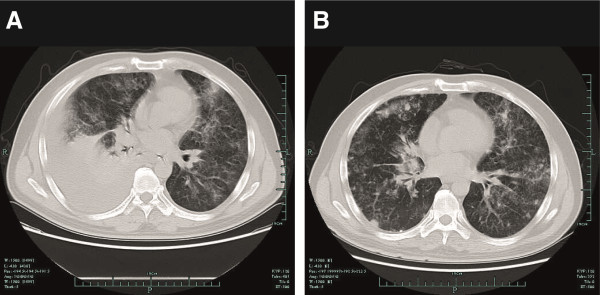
Computed tomography scans of the lungs. (A) Soon after the appearance of the patient’s respiratory symptoms, a computed tomography (CT) scan revealed septal thickening of the peribronchovascular interstitium, pleural effusion, segmental atelectasis in the right lower lobe of the lung and several enlarged lymph nodes in the mediastinum. (B) Discontinuation of anti-infection therapy, and 5 days after thoracentesis, extensive involvement of the parenchyma with septal thickening was evident, with reticulonodular densities in all lung fields.
